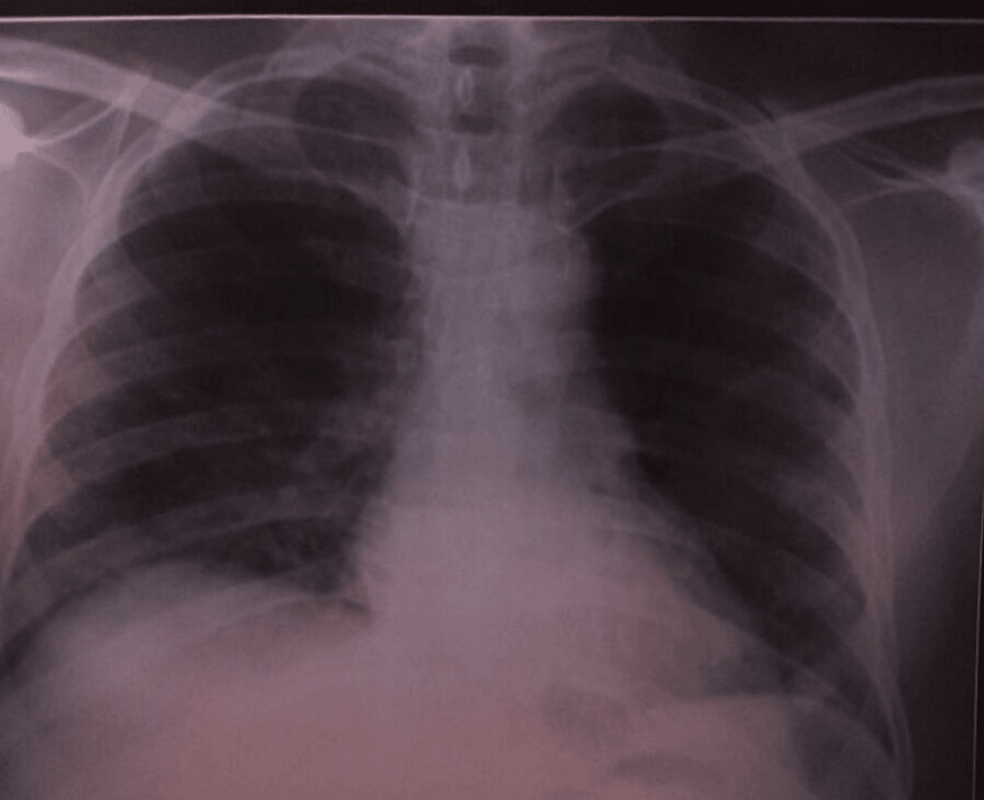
A chest X-ray (anteroposterior (AP) view) showing findings within normal limits
